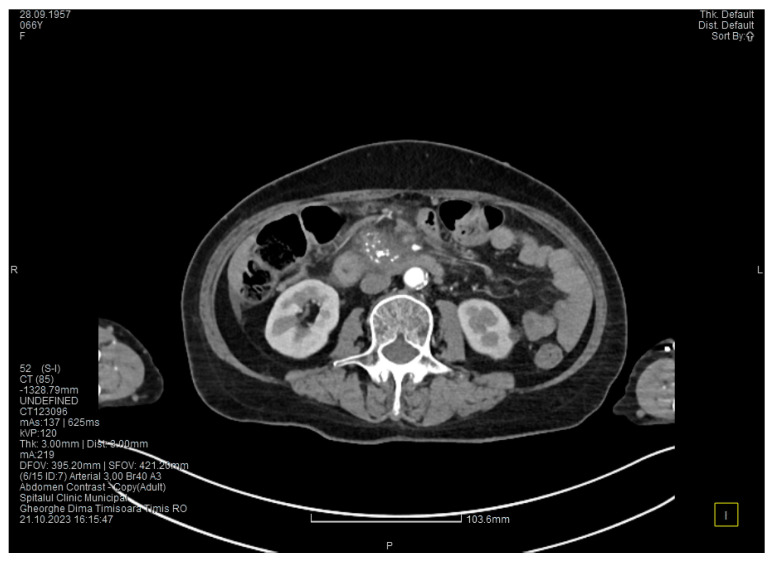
CT scan at the fourth episode of acute pancreatitis shows calcifications in the pancreatic head, thickening of the duodenal wall, and partial occlusion of the duodenum.
